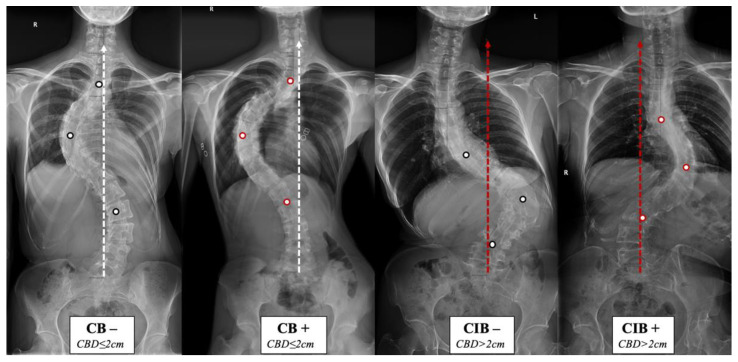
Coronal balance classification of adult idiopathic scoliosis according to the standing anterior-posterior whole spine radiograph. The apical vertebras distribution modifier (+/−) is introduced to supplement the previous classification based on the coronal balance distance (CBD). CB is diagnosed if CBD ≤ 2 cm, and CIB is diagnosed if CBD > 2 cm. The apical vertebra modifier is defined as negative (−) when CoAVs are on either side of the CSVL. The apical vertebra modifier is defined as positive (+) when CoAVs are on the same side of the CSVL. The white dots represent CoAVs, and the dotted arrows represent the CSVL.
